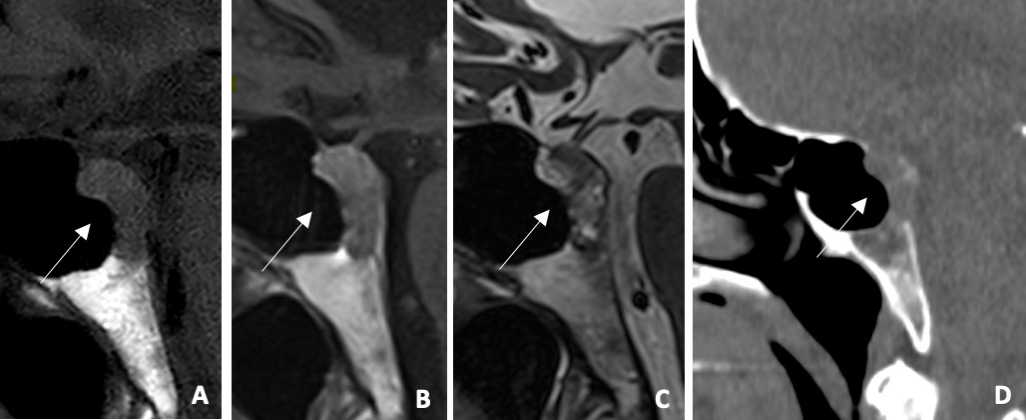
(A) Sagittal T1WI: hypointense expansive clival lesion (B) Sagittal post‑contrast T1WI: mild enhancement (C) Sagittal T2WI: heterogeneous lesion with hyperintense areas (D) Sagittal CT: Lytic clival lesion. Mildy enhancing clival lesion on MRI and lytic on CT.
